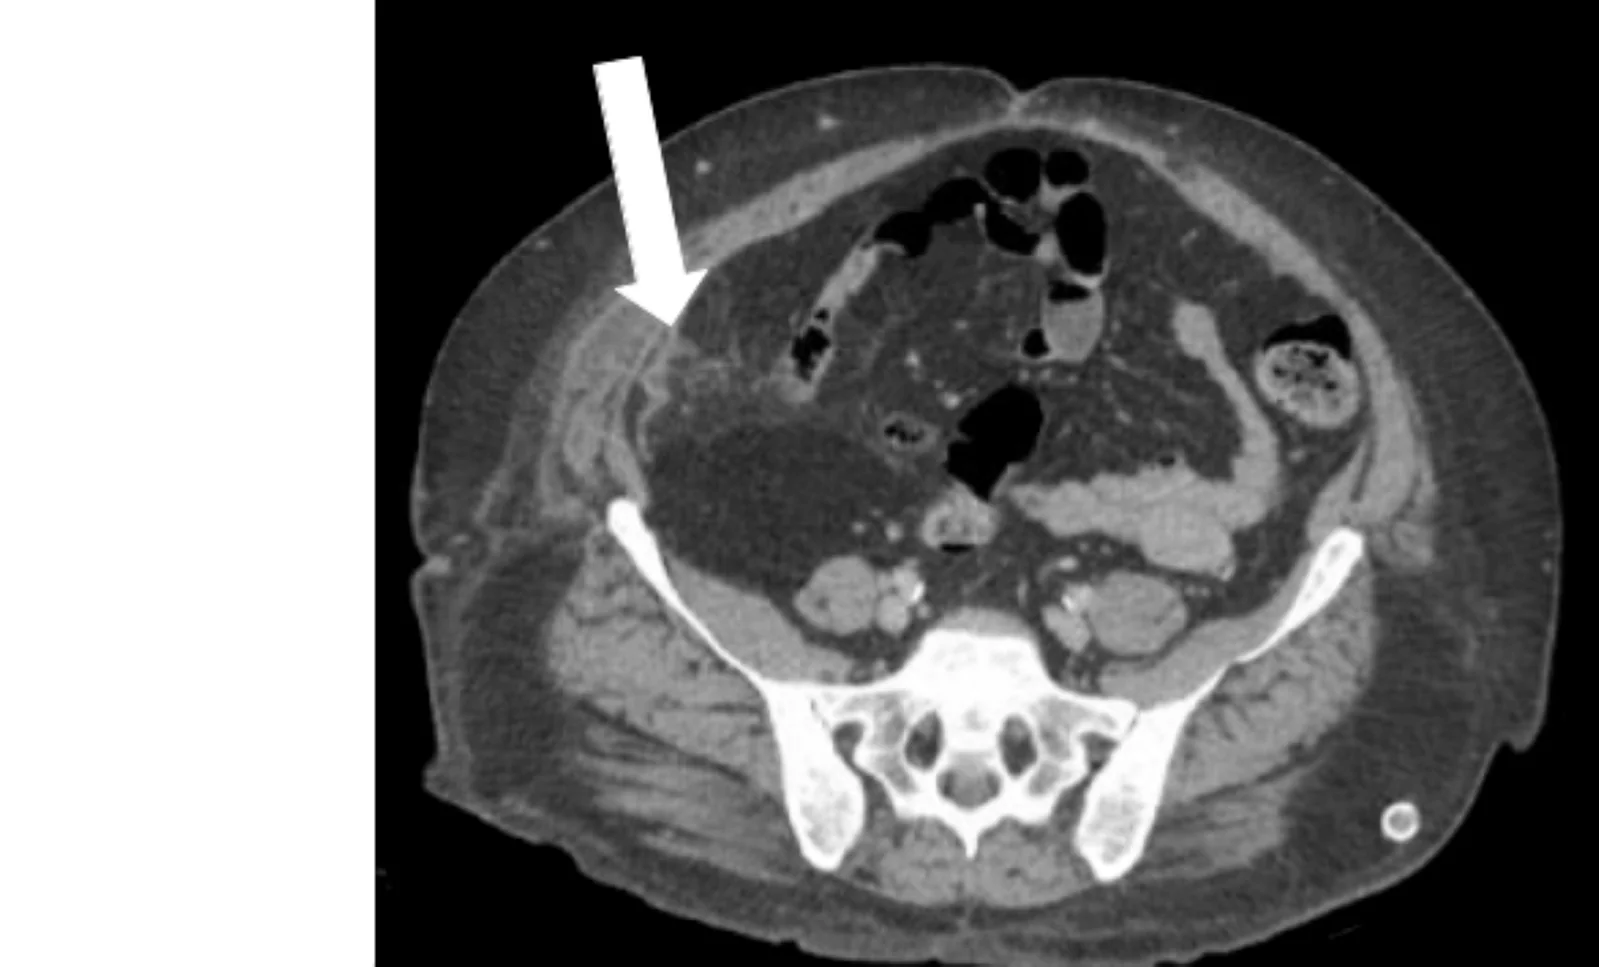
Postoperative CT of the Abdomen and Pelvis with Contrast Axial image obtained two weeks following appendectomy and Spigelian hernia repair with mesh, demonstrating a 3.7 cm superficial wound fluid collection (arrow) with surrounding inflammatory fat stranding. No internal gas, mature rim-enhancing capsule, deeper intra-abdominal extension, or radiographic evidence of communication with the underlying mesh is identified. These findings were concerning for an evolving early infectious collection rather than a mature drainable abscess.
